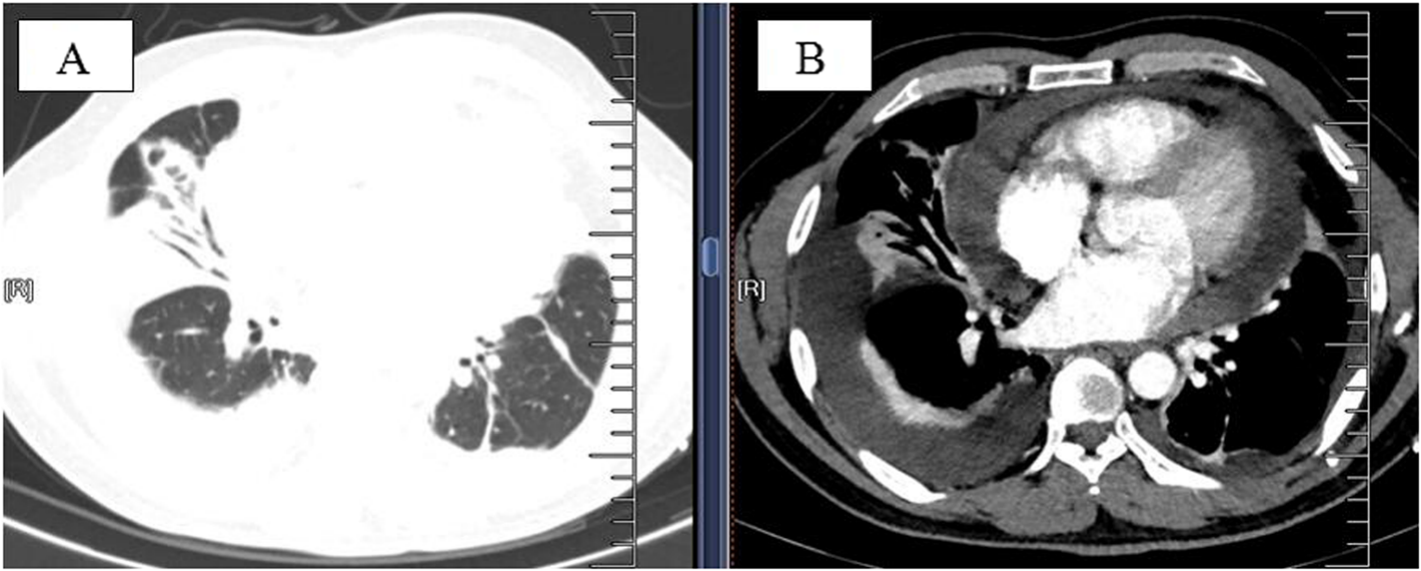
a 44-year-old male patient with IEPE (case 3). Chest CT a, b scans showed bilateral PE and consolidation in the lower right lung, pericardial effusion
